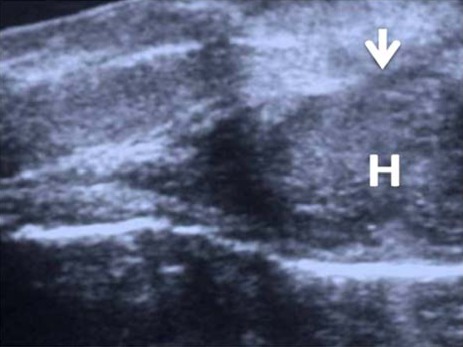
Longitudinal ultrasound image shows intracavernosal haematoma (H) and the site of ruptured tunica (marked by an arrow).
